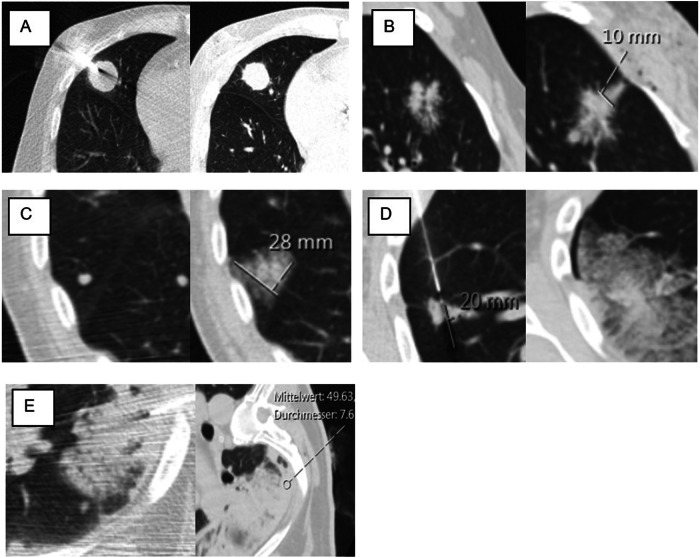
Pulmonary hemorrhage grading system. CT scan performed before/during (left) and directly after (right) demonstrate the following grades A Grade 0 without image morphological evidence of parenchymal hemorrhage after biopsy. B Grade 1: new linear, fine ground glass with a width under 2 cm can be delineated in the access route after the biopsy. C Grade 2: after the biopsy, the target lesion can no longer be demarcated if the alveolar hemorrhage is locally larger than 2 cm. D Grade 3: lobar hemorrhage and the biopsy channel before. E Grade 4: hemaothorax with immediate hyperdense fluid within the pleural cavity after biopsy. Mittelwert, mean; Durchmesser, diameter; mm, millimeter
